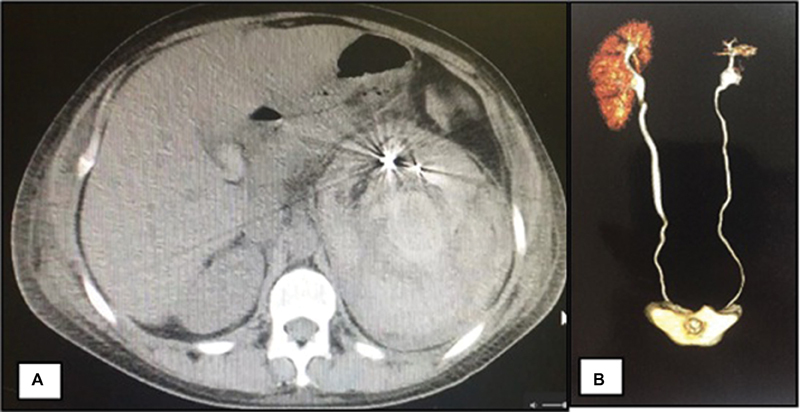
(A) Axial section of the computed tomography scan in the third postoperative day of the embolization demonstrating asymmetry between the right and left renal contours due to the massive perirenal hematoma on the left; the artifacts with metallic density correspond to the coils used in the embolization; (B) urotomography in the tenth postoperative month demonstrating the remaining functional left kidney parenchyma.
